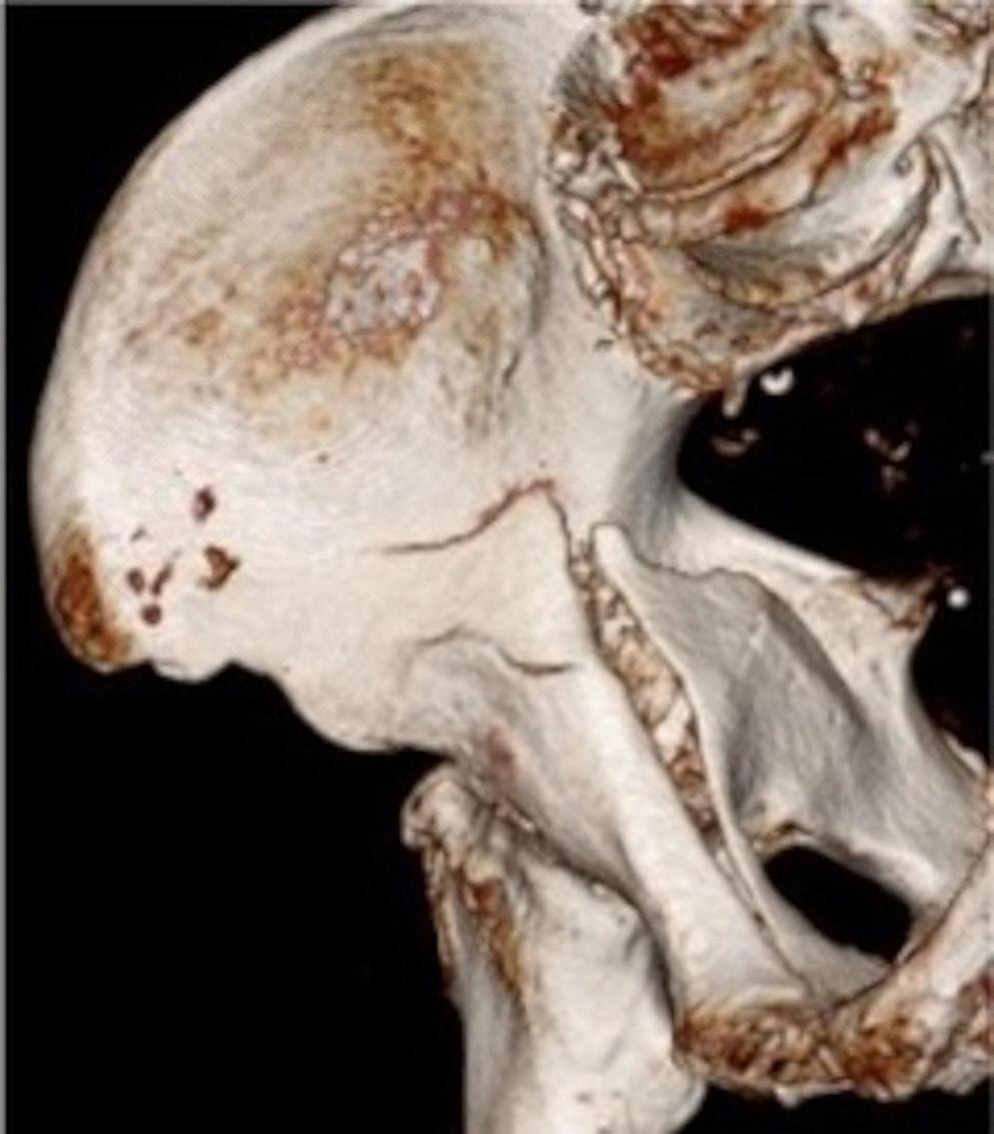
3-D CT reconstruction of an anterior column posterior hemitransverse acetabular fracture (ACPH)
